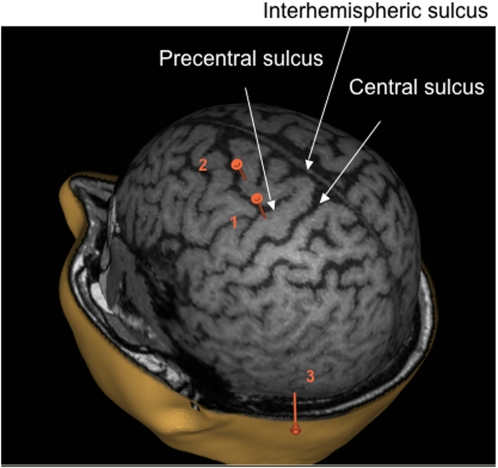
MRI reconstruction of the three-dimensional brain volume for one subject. Three sites were targeted : (1) the primary hand motor area, (2) the premotor area and (3) a control site lying in the temporo-occipital region.
